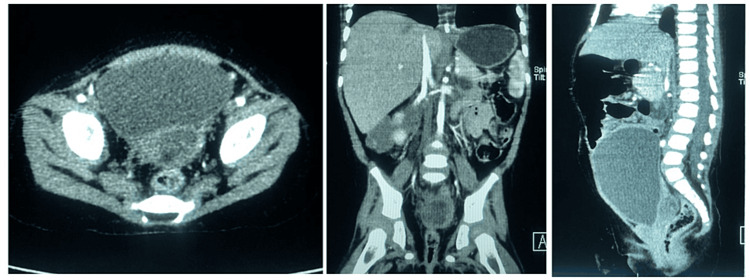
CT scan after chemotherapy. Abdominopelvic CT after four cycles of chemotherapy showing a decreased size of the mass. It appears heterogeneous with an irregular hypodense center and is located in the rectovesical septum, measuring 18x27 mm in the axial plane. There is no evidence of adenomegaly in the common iliac chains.
